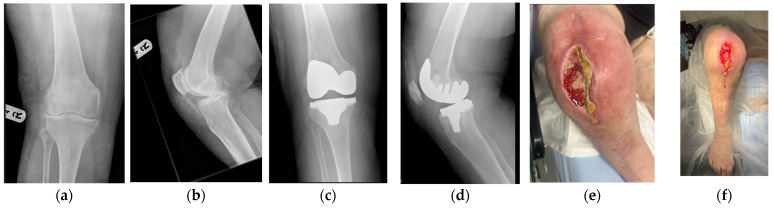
(a–d) Case 4 radiographic findings at presentation. (e,f) Case 4 clinical presentation.
